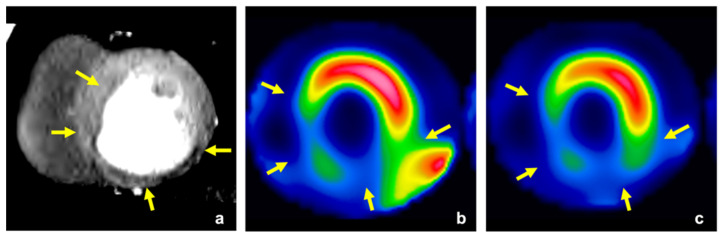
A 58-year-old man with effort angina. Stress CTP image of the left ventricle showed extensive myocardial perfusion abnormalities in the inferior, septal, and lateral inferior wall (yellow arrow, (a)). The perfusion abnormalities were detected with MPR (0.50 < cut-off value: 0.81), but not detected with TPR (0.96 > cut-off value: 0.92). SPECT ((b), stress; (c), rest) showed fixed perfusion abnormalities in these lesions, which indicated extensive old myocardial infarction (yellow arrow). CTP, computed tomography perfusion; MPR, myocardial perfusion ratio; TPR, transmural perfusion ratio; SPECT, single-photon emission computed tomography.
